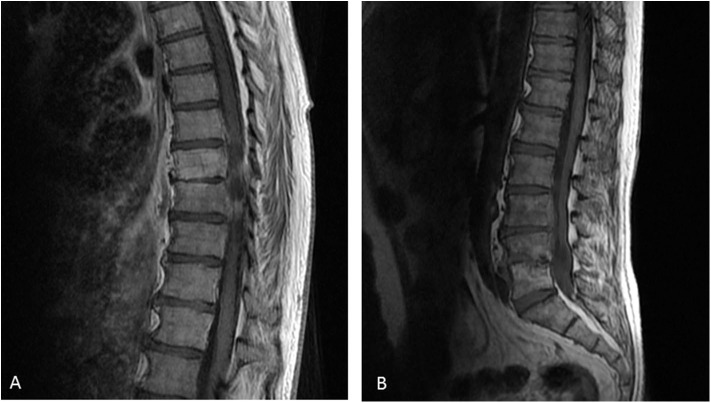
Vertebral magnetic resonance imaging of the vertebral lesions. Vertebral magnetic resonance imaging revealed lesions of the vertebral bodies at T8-T9-L4-L5-S1 and invertebral disks between T8-T9 (A) and L4-L5-S1 (B), with an epidural abscess of 5 cm at the L3 and L4 levels, consistent with a pyogenic vertebral osteomyelitis.
